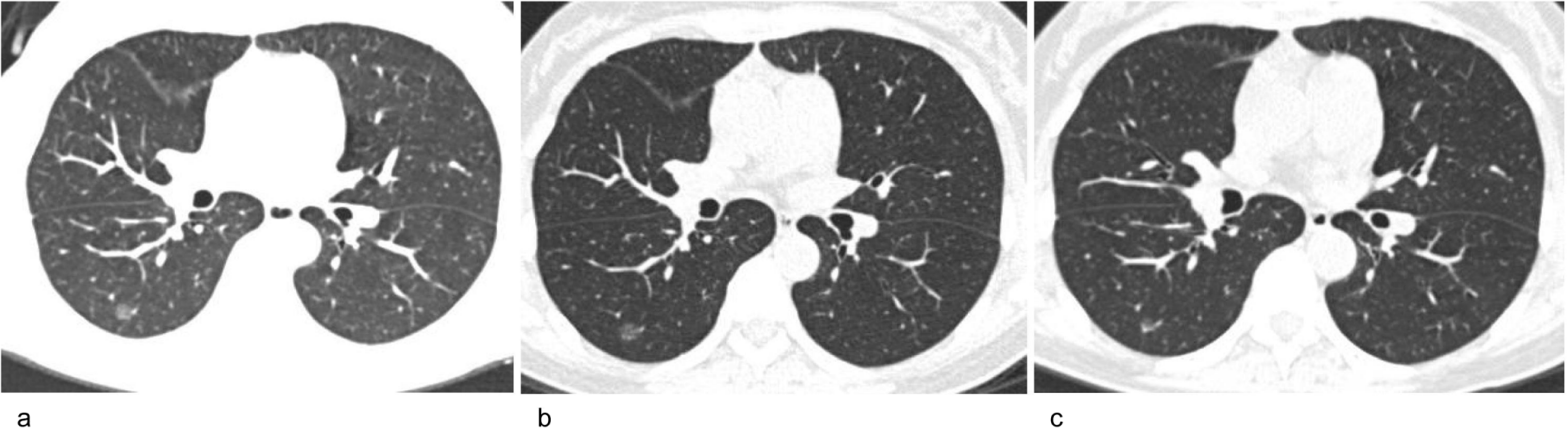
A 49-year-old woman with subsolid nodule growth after long-term follow-up CT. a Baseline CT. Transverse plain CT of a pure ground-glass nodule in the right lower lobe. The nodule size (longest diameter) was 8 mm on transverse CT images. b Follow-up CT obtained 5 years after baseline. The pure ground-glass nodule was stable. c Follow-up CT obtained 6 years after the baseline CT. A new solid component appeared in the nodule, and its size decreased from 8 to 6 mm. The growing nodule was a minimally invasive adenocarcinoma, as confirmed by pathology
